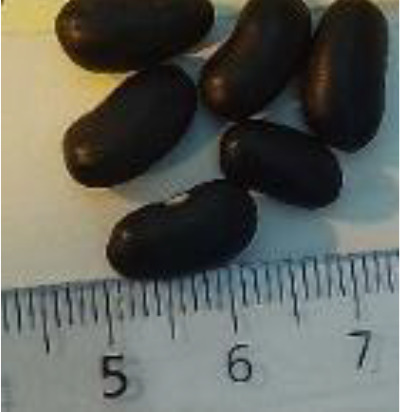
DF, Days to flower; DPM, Days to physiological maturity; Pod_P, pods per plant; Seed_P, Seeds per plant; S_pod, Seeds per pod; PYLD, Yield; CT, Cooking time; Wt_100S, 100-seed weight. The superior performing genotypes were selected based on those that had a superior BLUP value than the average BLUP for each trait across all the traits.
